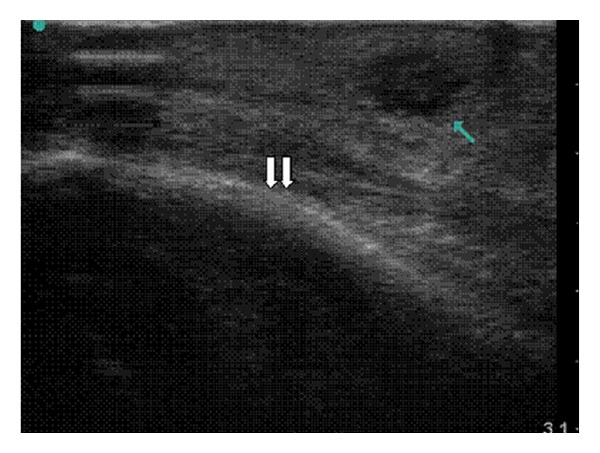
Bedside ultrasound of case patient using linear probe, sagittal view. A distinct abscess (single arrow) is apparent in the subcutaneous tissue overlying the maxilla (double arrows). The single arrow points to the abscess, which is distinguished as a well-circumscribed area of hypoechoic fluid.
